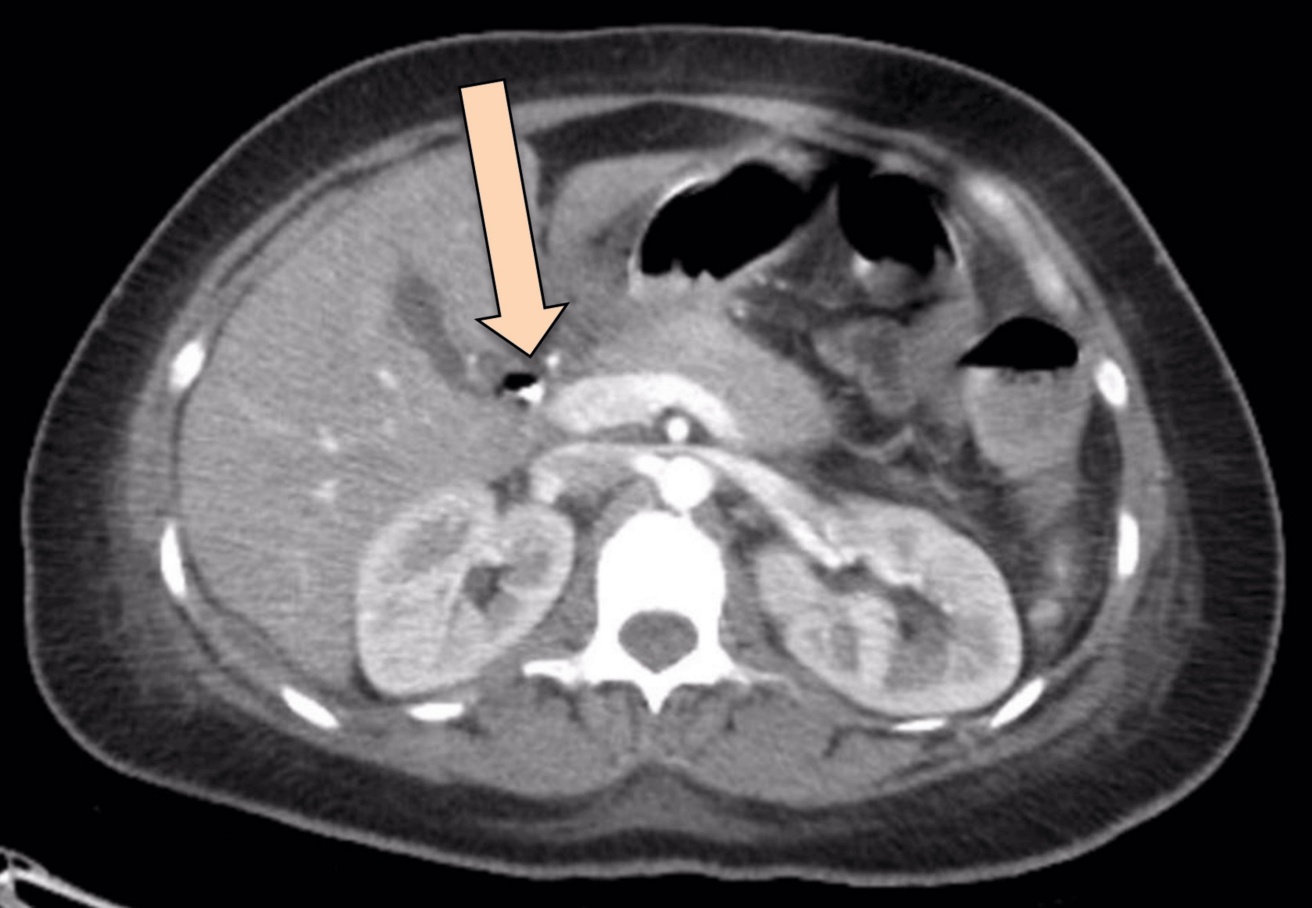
Follow-up axial view CT abdomen with IV contrast The arrow shows persistent communication between the para-duodenal collection and the posterior aspect of the distal CBD CT: computed tomography; IV: intravenous; CBD: common bile duct
